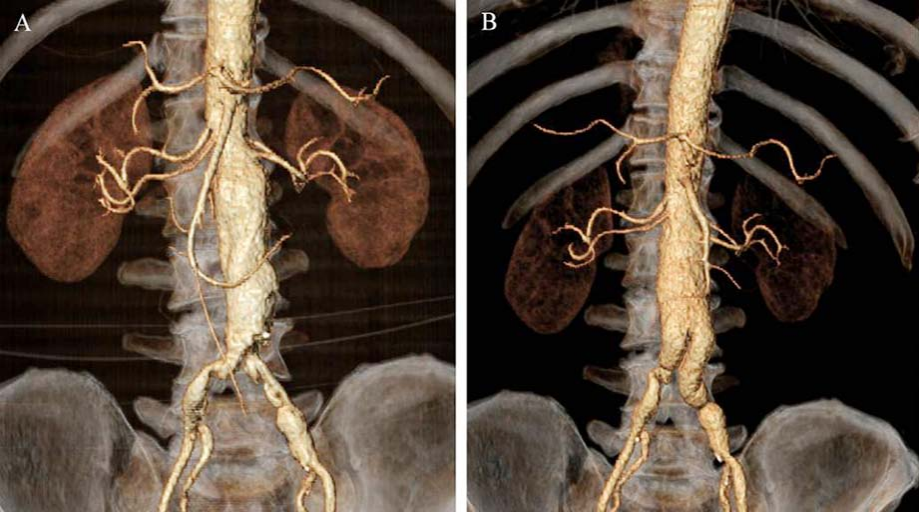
CT images for one patient who received traditional surgery. A: Preoperative CT discloses AAA; B: Re-examination in the postoperative 6th month shows unobstructed artificial blood vessels, proximal-end abdominal aorta anastomotic stoma and distal-end common iliac artery anastomotic stoma.
